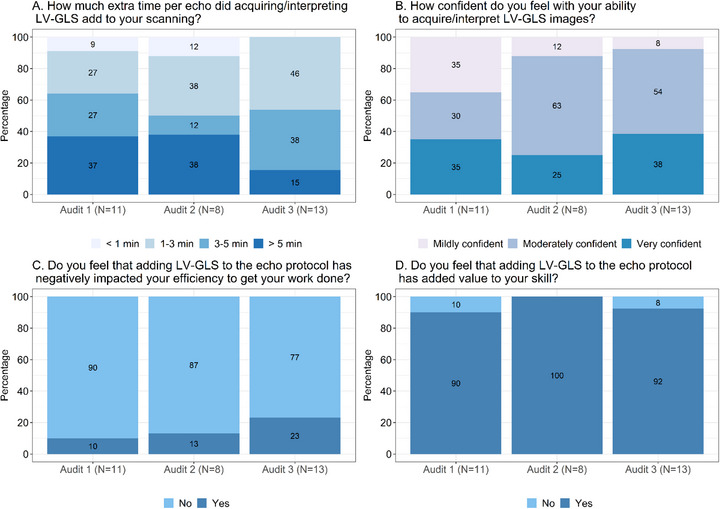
Survey administered after each audit to sonographers acquiring left ventricular global longitudinal strain. Results from the surveys administered to sonographers acquiring LV‐GLS after each audit are shown in this figure using stacked bar charts with components' proportional contributions. The survey contained four key questions (A, B, C, and D), and sonographers’ responses were compared from audits 1 through 3 for each question. The number of sonographers participating in the surveys at each audit is indicated.
